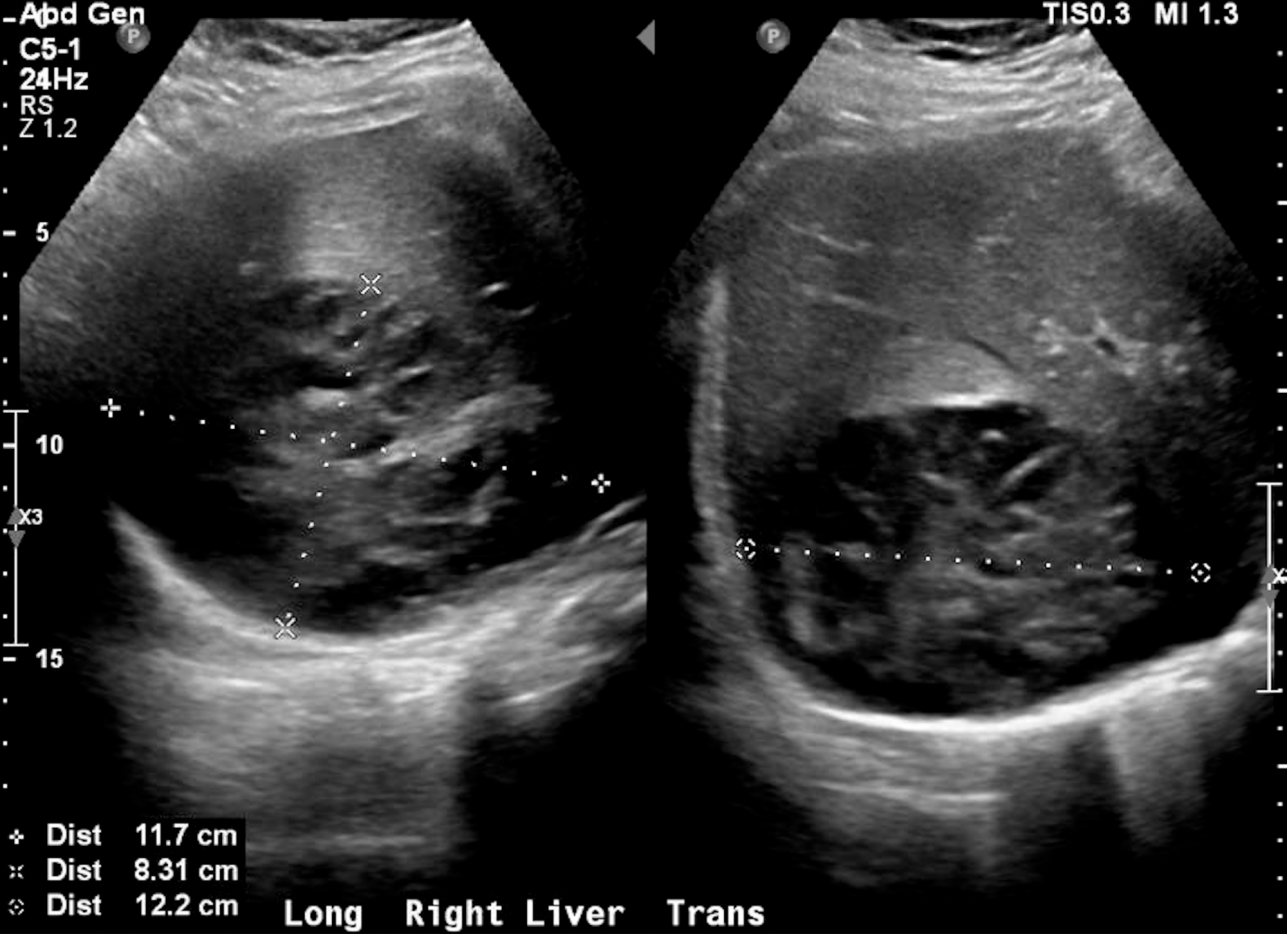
Ultrasound of the liver A right upper quadrant ultrasound revealed a large right hepatic complex solid and cystic mass measuring 11.7 cm x 12.2 cm x 8.3 cm, consistent with computed tomography findings.
